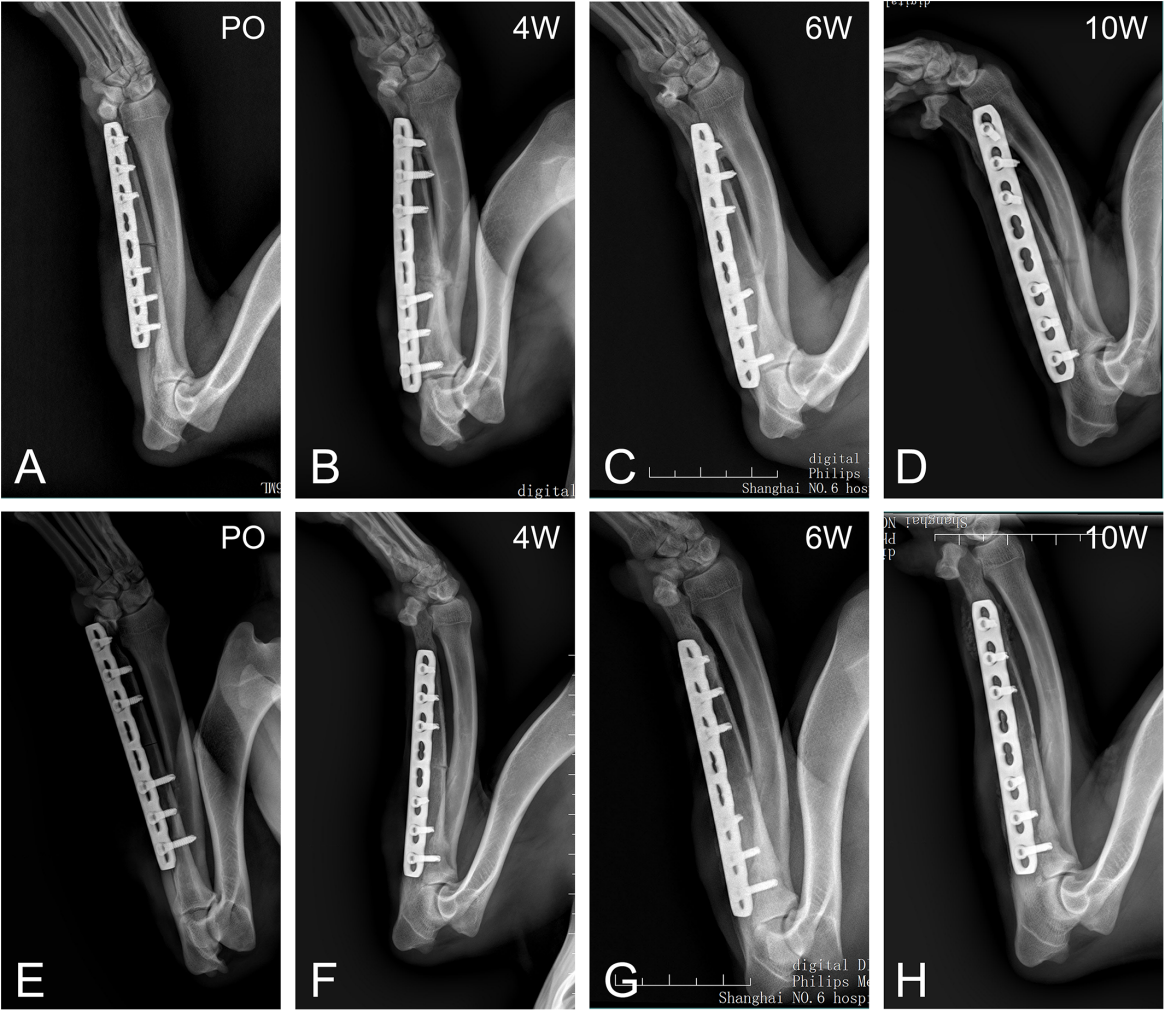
1 day, 4, 6, 10 weeks postoperative radiographs images for beagles treated with minimally invasive plate osteosynthesis (MIPO) (A-D) and open reduction internal fixation (ORIF) (E-H).
